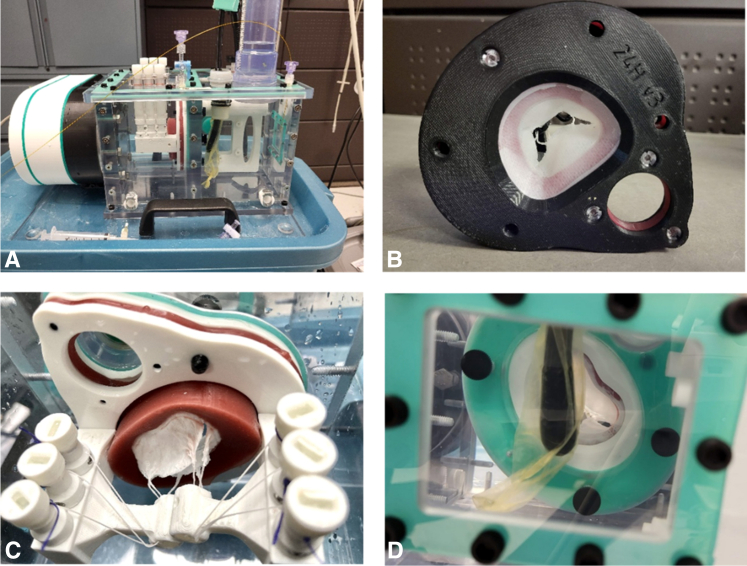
Silicone valve replica viewed in pulse-duplicator from above with chordae tensioners (A), and from atrial en face view (B). Pulse duplicator setup from side (C) and from en face view (D), including the transesophageal echocardiography probe used for evaluation.
